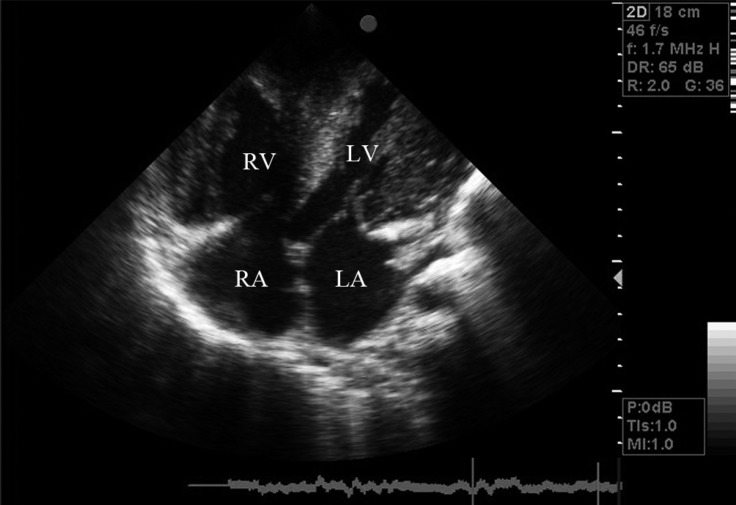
4-chamber view in echocardiography showed severe LV hypertrophy indicated hypertrophic cardiomyopathy.
